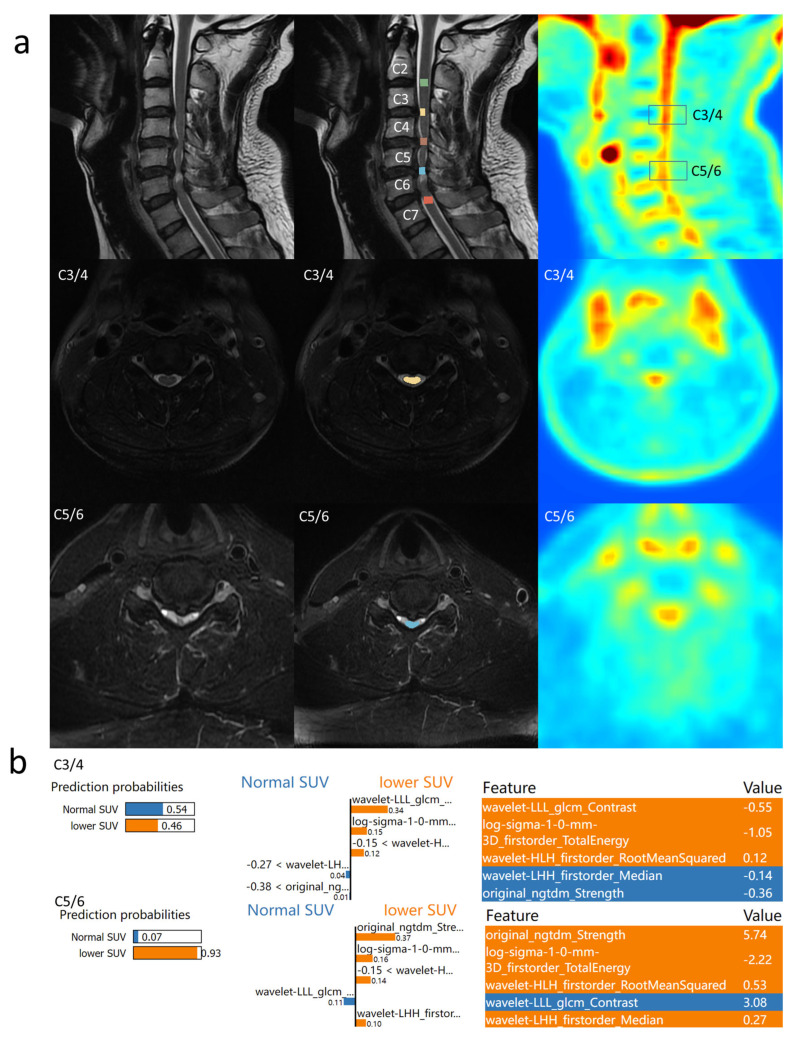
Prediction result of Example 1: (a) the PET/MR results of Example 1 are shown. Cervical spinal cord segments from C2 to C7 and five intervertebral disc segments were labeled. SUV_max_ was normal at the C3/4 level and SUV_max_ was decreased at the C5/6 level; (b) the LIME results of the proposed model are shown.
